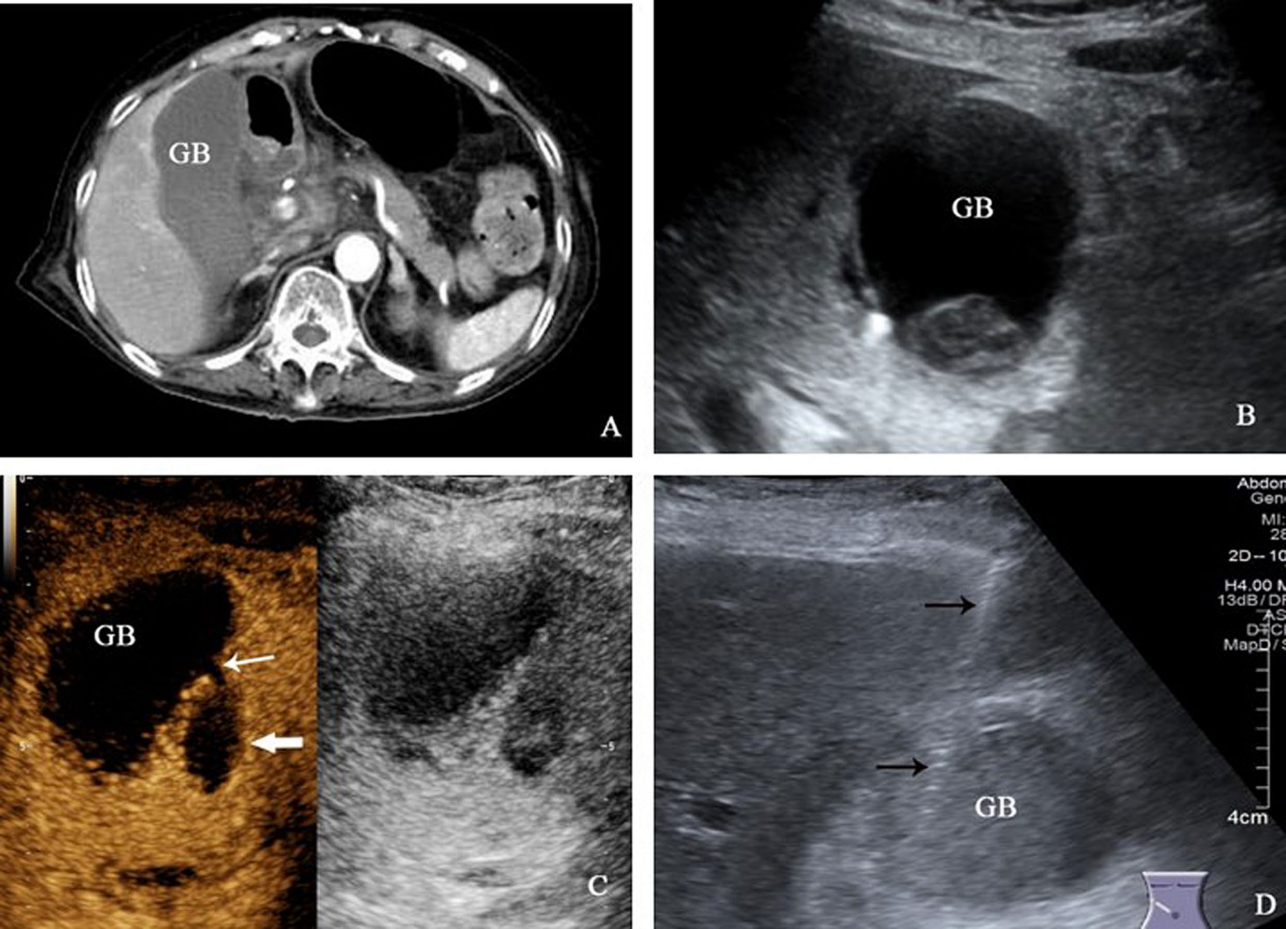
(A) The contrast-enhanced CT examination revealed a significant enlargement of the gallbladder, accompanied by the presence of low-density exudation shadow around it. (B) The gallbladder exhibited a transverse diameter of approximately 4.5 cm, with thickening of the gallbladder wall. Additionally, gallstones and viscous bile were observed within the cavity. (C) CEUS displayed a discontinuity in the left lateral wall of the gallbladder, with a local interruption of approximately 2 mm (thin white arrow), and an unenhanced area of 2.3 × 1.5 cm was visible beside the break (thick white arrow). (D) Percutaneous transhepatic gallbladder puncture under ultrasound guidance was performed, and the puncture needle was shown by thin black arrow.
